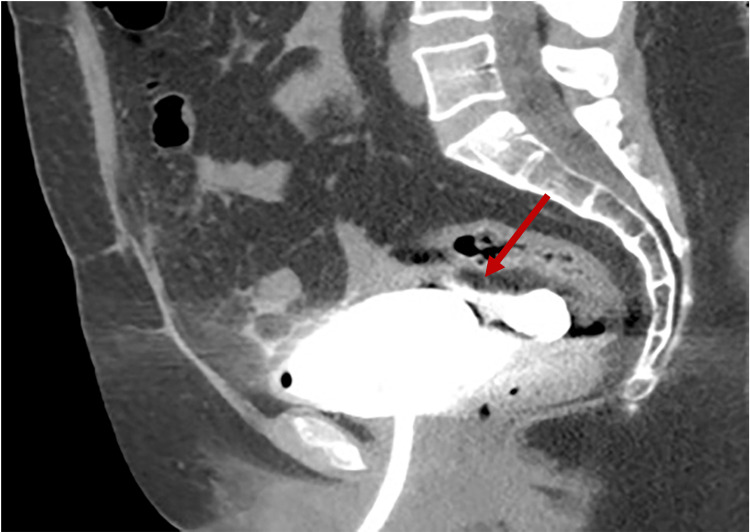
Sagittal CT cystography image of the bladder diverticulum rupture, with urine extravasation.
